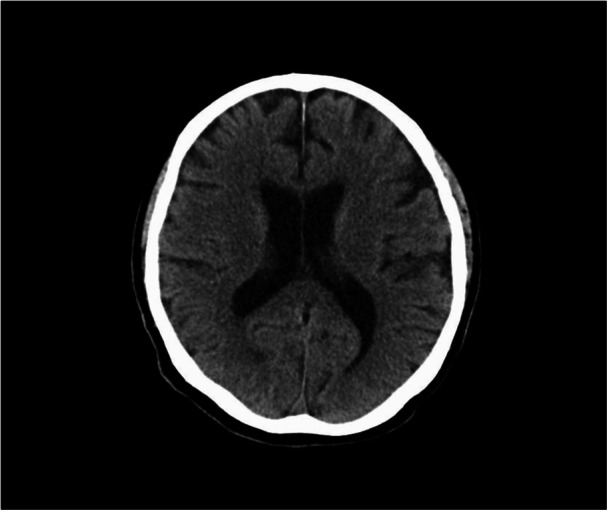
Head computed tomography (CT) on the day of consultation to the psycho‐oncology department, showing no brain metastasis, hemorrhage, and hydrocephalus.
